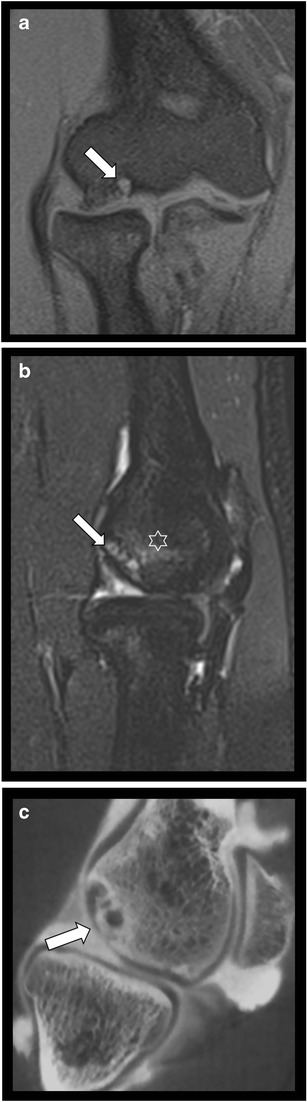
Osteochondral lesion of the capitulum. a. Coronal T1-WI fat saturated MR image showing a osteochondral lesion of the capitulum. b. Sagittal PD MRI fat saturated image at the level of radio-humeral joint revealing subchondral bone marrow changes (star) and subchondral cyst formation (arrow), the cartilage cannot be evaluated precisely. c. Sagittal reformatted CBCT-A at the level of the radio-humeral joint showing the osteochondral lesion of the capitulum with subchondral cyst formation, surrounding sclerosis and subtle focal thinning of the articular cartilage (arrow)
